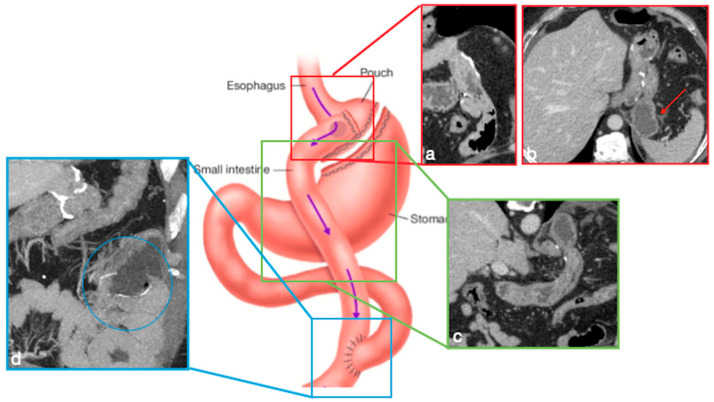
Gastric bypass. Surgical sutures seen at CT. Gastric pouch with gastrojejunostomy (a,b axial oblique view) and excluded stomach (b, arrow). Roux limb (c, coronal oblique view) of length of 75 to 150 cm from the jejunal division point for an average of 120 cm, jejunojenuostomy (d, circle, coronal view) between the biliopancreatic limb and the distal segment of jejunum (roux limb). The scheme was adapted from https://www.uptodate.com/contents/image/print?imageKey=GAST%2F79256 (accessed on 23 October 2022).
